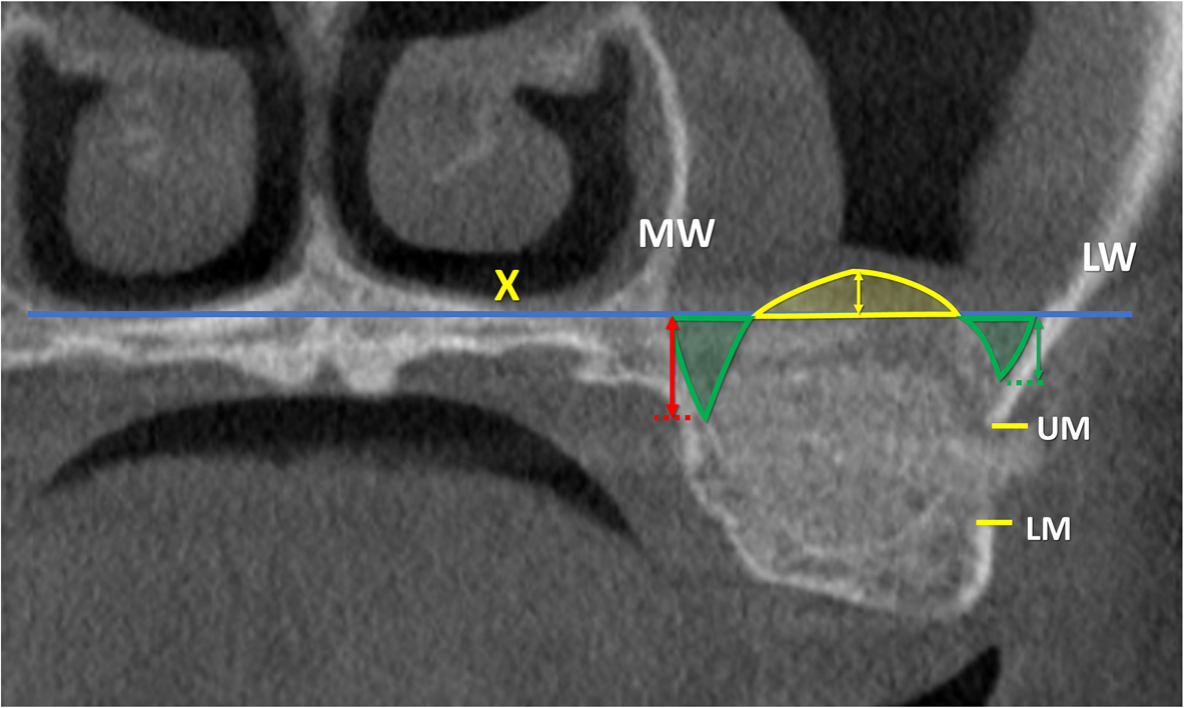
T1, coronal view of a no-membrane CBCT; X, line drawn following the floor of the nose; F, base of the sinus floor; MW, medial wall of the sinus; LW, lateral wall of the sinus; UM, upper margin of the antrostomy; LM, lower margin of the antrostomy. Exceeding area (bordered in yellow), area above the line X filled with biomaterial/ bone tissue. Residual areas (bordered in green), the areas below the line X not filled with biomaterial/ bone tissue. Floor augmentation heights at the lateral (green arrow), middle (yellow arrow), and medial (red arrow) aspects
